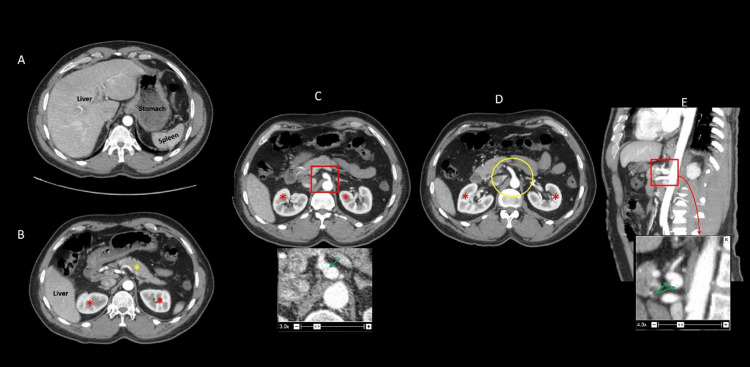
Computerized tomography (CT image). (A, B): Axial images with apparently normal-appearing liver, stomach, spleen, pancreas (yellow asterisk), and kidneys (red asterisk). (C) Axial image depicting the bulbous dilatation of the celiac artery trunk, which upon magnification demonstrates the intimal flap (green arrow). (D) Axial image showing the normal origin of the superior mesenteric artery. (E) Sagittal image remonstrating the dilated celiac artery trunk with an intimal flap (green arrow), while the normal superior mesenteric artery is visualized below.
